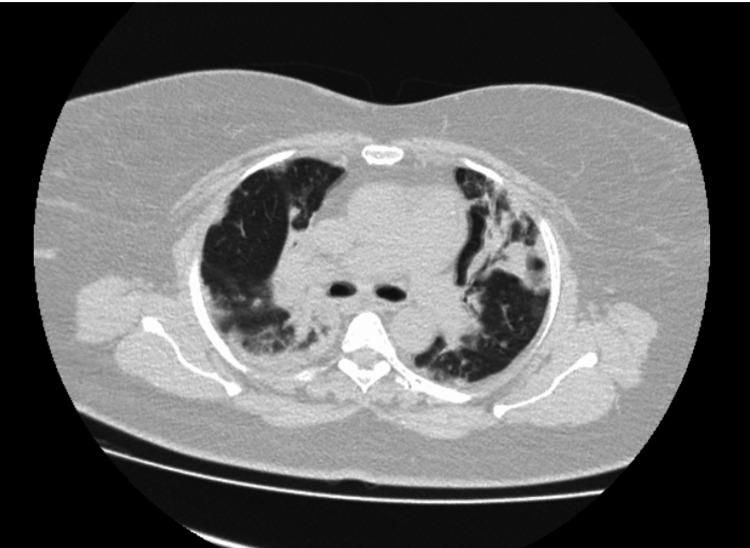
Chest CT showing bilateral consolidation on both lungs on admission CT: computed tomography
